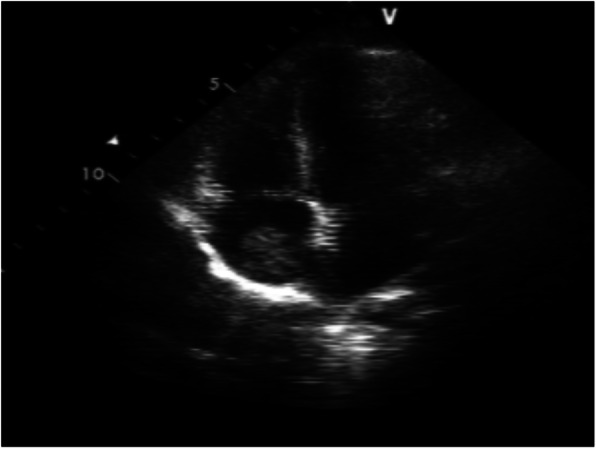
Echocardiography:right atrial thrombus
